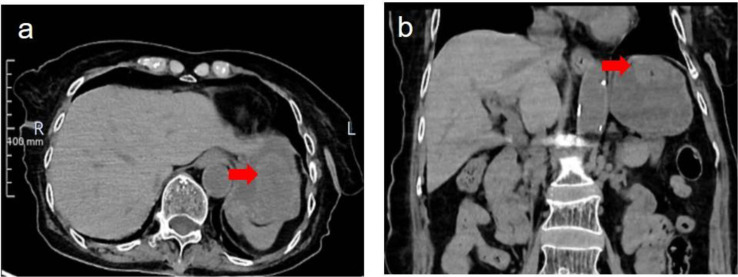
Intraluminal tumor in the gastric funds on CT. Axial (a) and coronal (b) CT images reveal an intraluminal gastric fundus tumor with well-defined borders (arrow).
